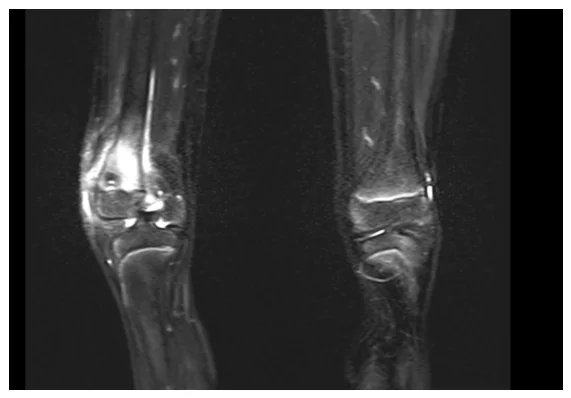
MRI imageof the right knee in a patient with CNO without bone destruction. Bone edema, a hyperintense area of 1.5 × 0.8 cm with a lower signal area around it, and a periosteal reaction in the distal metadiaphysis of the femur. Lateral and dorsal soft tissue swelling.
